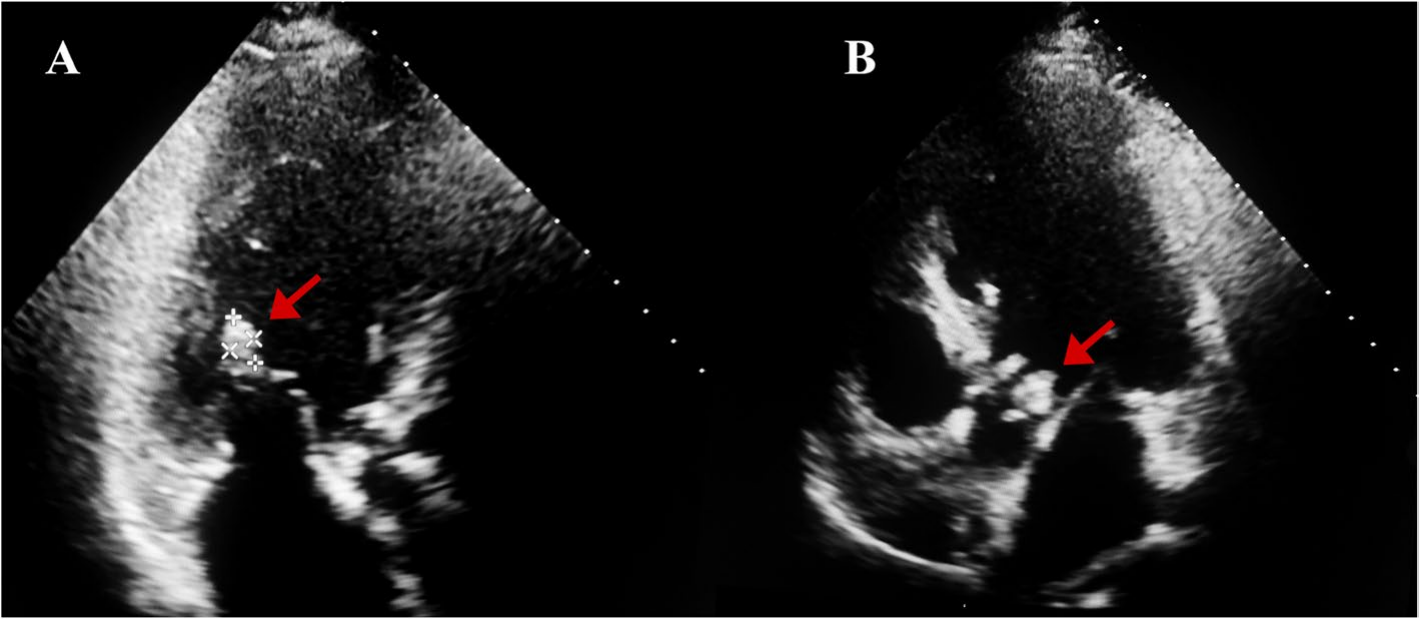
Transthoracic echocardiogram showing multiple vegetations. A The representative vegetations (arrow) in the mitral valve. B A 9 mm × 6 mm vegetation (arrow) in the aortic valve
